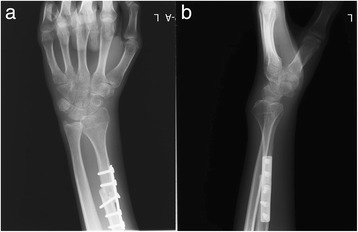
Anteroposterior and lateral X-ray images of the wrist taken 3 months after the reconstruction (a, b)
